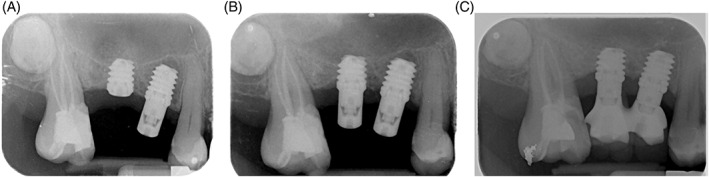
Intraoral radiographs of one of the patients included in the present research: at T0 immediately after implant insertion (on the left); at T3 (in the middle) and at T12, 1 year after implant insertion (on the right)
